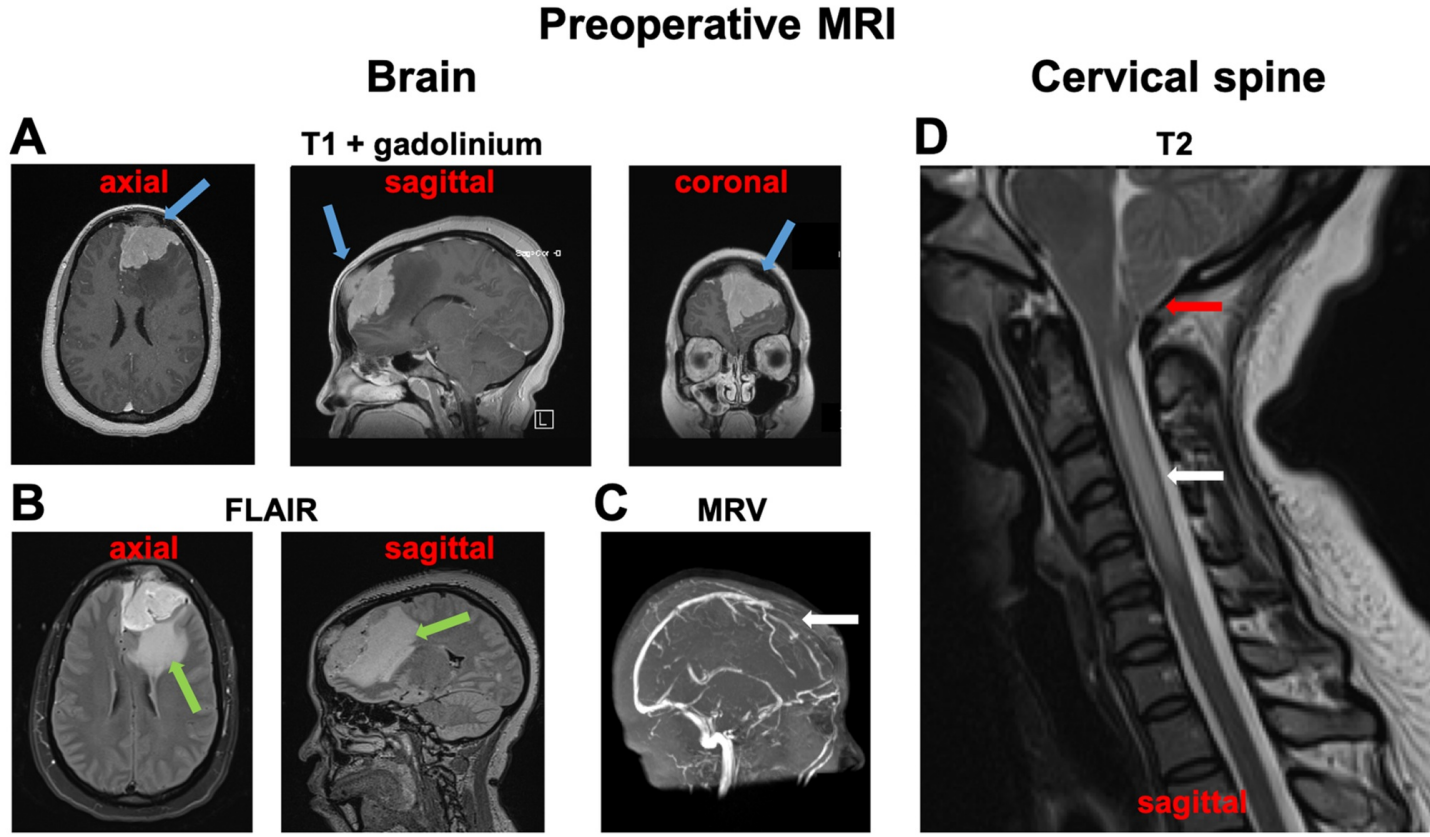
Preoperative MRI imaging A. Post-gadolinium T1-weighted images of the tumor (blue arrows). Note the calvarial involvement. B. Fluid-attenuated inversion recovery (FLAIR) imaging shows extensive peritumoral edema (green arrows). C. MR venogram (MRV) showed the occlusion of the superior sagittal sinus at the level of the tumor (white arrow). D. Sagittal T2 imaging of the cervical spine demonstrates tonsillar herniation (red arrow) below McRae’s line and cervical syringomyelia (white arrow).
